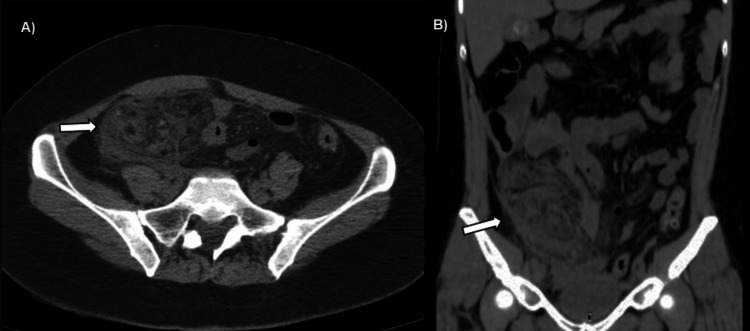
Computerized tomography in a simple phase of the abdomen. Axial (A) and coronal (B) CT scans show a rounded, partially defined, and heterogeneous mass with fat density localized to the right lower quadrant, adjacent to the anterior abdominal wall (thick arrow).
